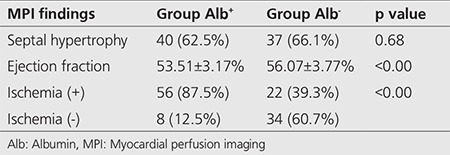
Myocardial perfusion single-photon emission computed tomography findings
